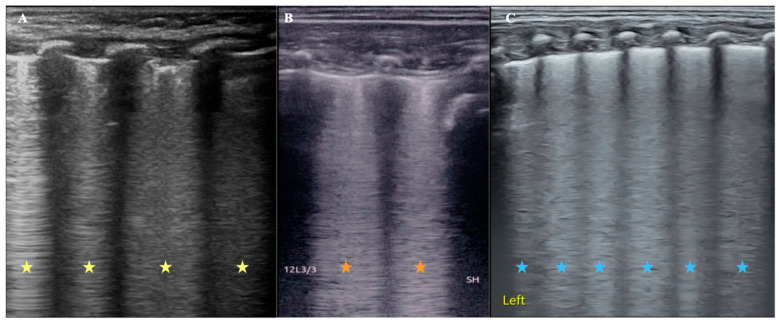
Comparison of ultrasound B-line manifestations of pulmonary edema with different properties. (A): An infant with viral pneumonia. LUS appears as confluent B-lines (yellow asterisk). (B): Rabbit model of pulmonary edema. Because LUS examination was performed immediately after 0.9% NaCl was injected into the lung from the endotracheal tube, the kind of pulmonary edema should be mainly alveolar pulmonary edema at this condition, and LUS appeared as confluent B-lines (brick-red asterisk). (C): Infant with wet lung. The mixed pulmonary edema and LUS also showed confluent B-lines. It is difficult to distinguish the type of pulmonary edema from the appearance of the B-lines (blue asterisk).
